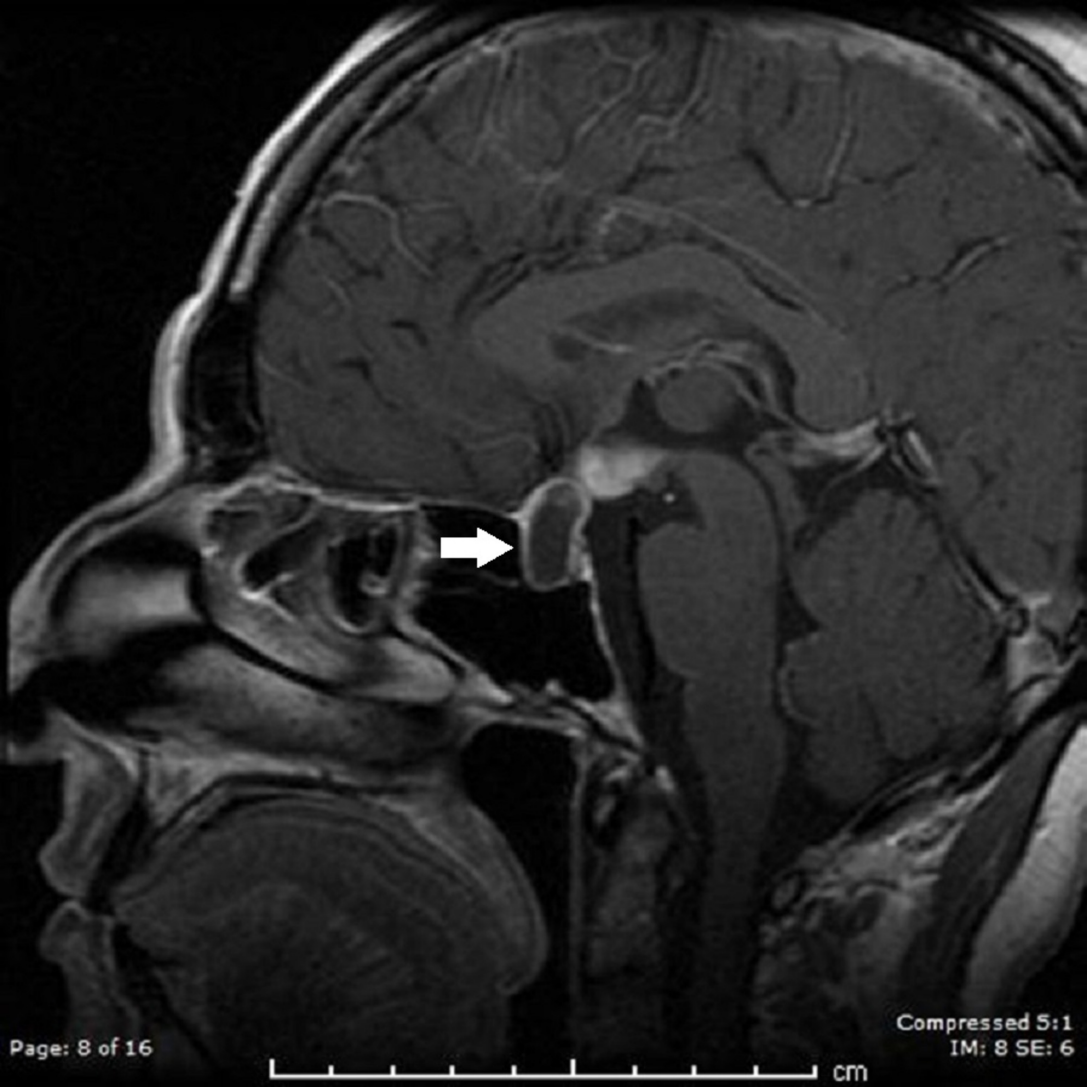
Post-contrast sagittal T1 magnetic resonance imaging on initial admission, showing large parasellar cystic mass
